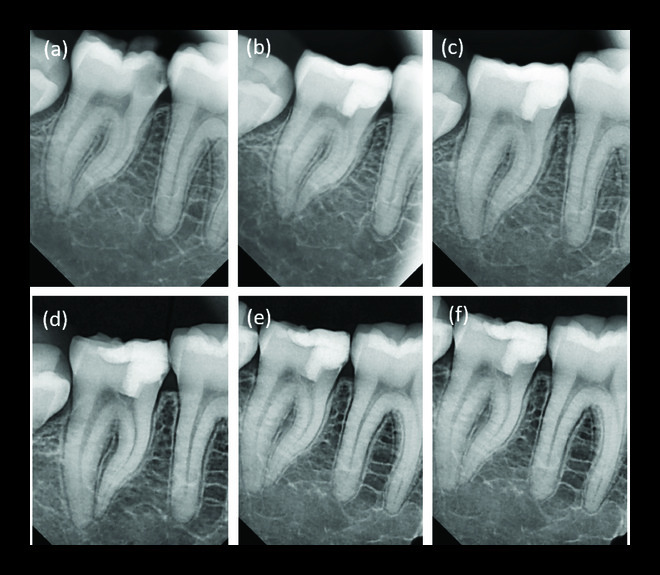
(a) Diagnostic periapical radiograph. (b) After partial pulpotomy. (c–f) One-year, two-year, three-year, and four-year postoperative periapical radiographs, respectively, showing normal periapical tissues and mineralized bridge formation.
